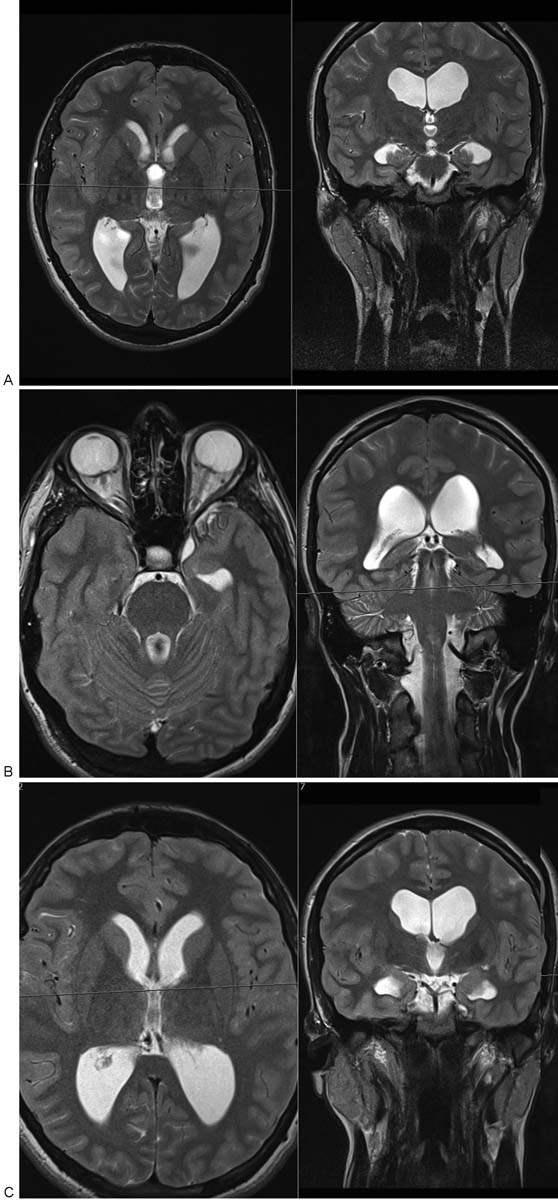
(A, B, C) T2-weighted magnetic resonance imaging of the brain in the second case demonstrates nonobstructive hydrocephalus with enlargement of all four ventricles.
